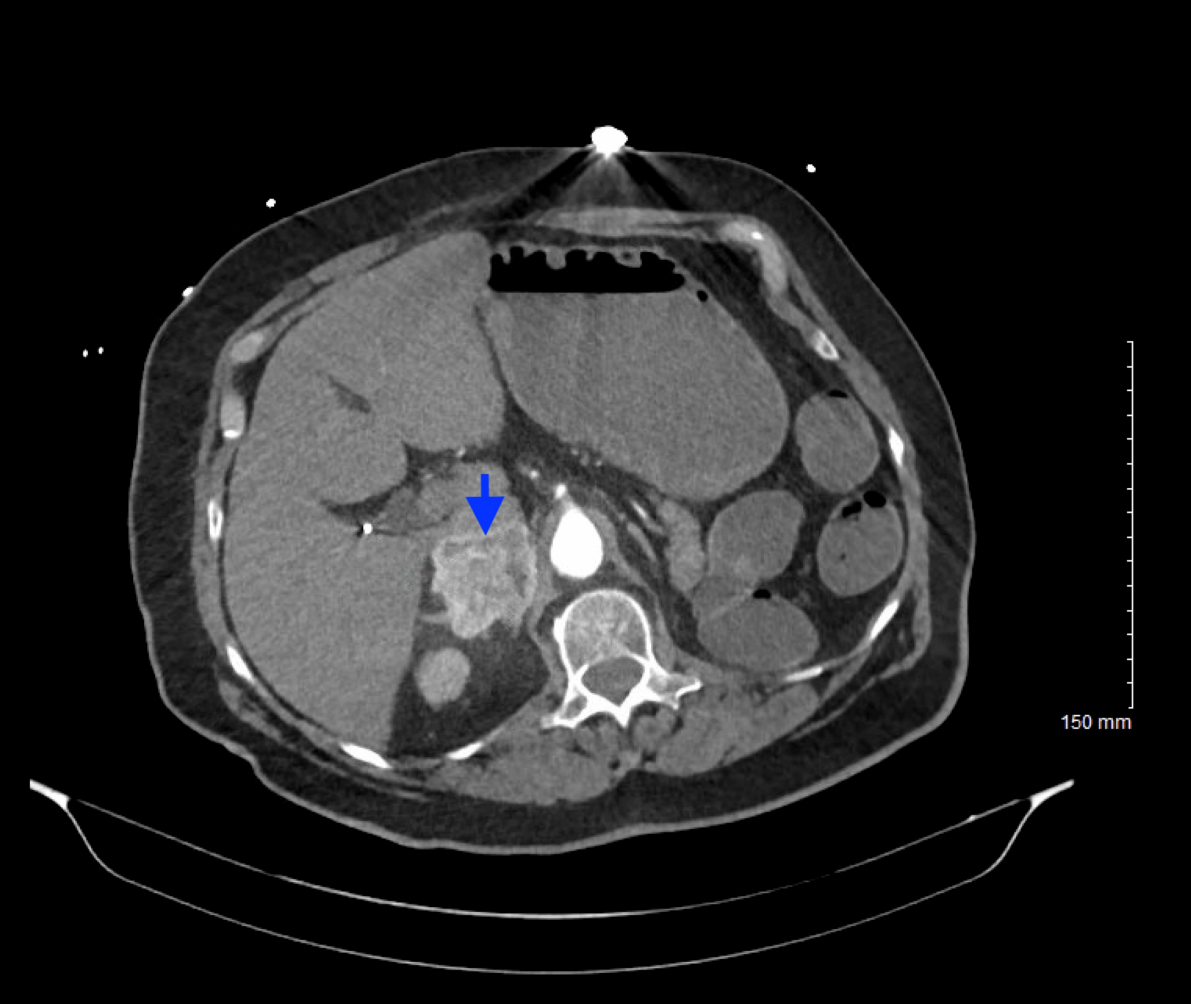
CT abdomen/pelvis with IV contrast (axial view) CT shows an arterial enhancing mass lesion (blue arrow) in the right retroperitoneal space measuring 4.2 x 4.3 x 5.5 cm.
